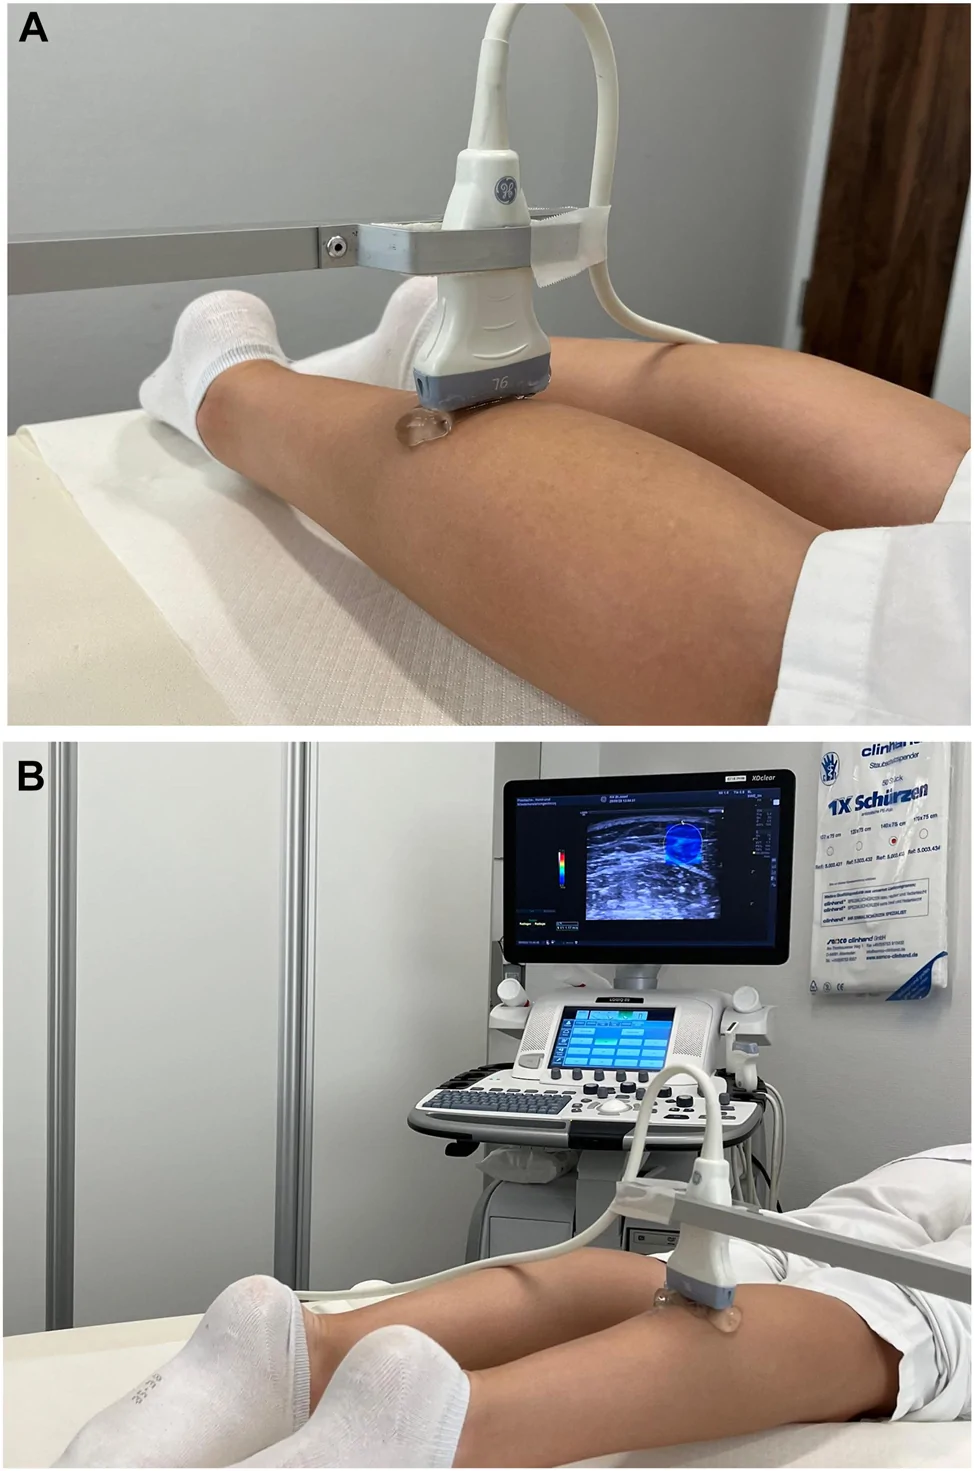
Patients and transducers positioning during elastography. (A – B) exemplary positioning of a patient during elastography assessment in prone position. Standardized placement of the ultrasound transducer at predefined anatomical landmarks (thigh and calf) and after lymphovenous anastomosis (LVA). The fixed orientation of the transducer and minimal compression technique were used to reduce operator-dependent bias.
